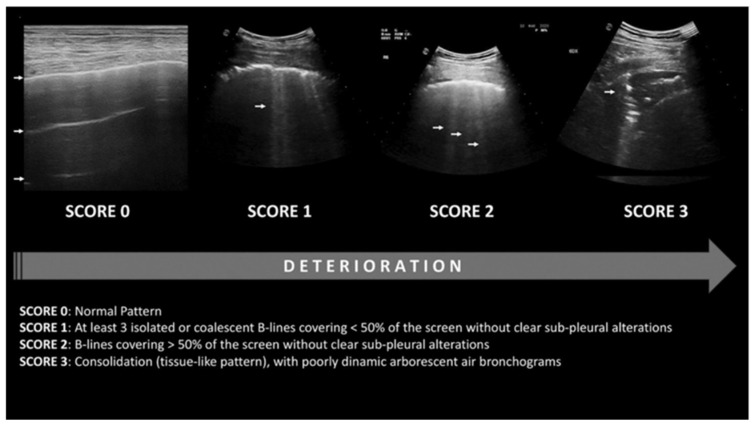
Lung finding for each lung ultrasound score with 12 lung fields. Lung regions exhibiting normal aeration (N) are assigned a score of 0 (white arrows with score 0 denoting A lines); moderate aeration loss (B1) receives a score of 1 (white arrows with score 1 denoting B lines); severe aeration loss (B2) is given a score of 2 (white arrows with score 2 denoting B lines covering > 50% of the screen); and full aeration loss (consolidation; C) is allocated a score of 3 (white arrows denoting consolidation). The cumulative readings for all 12 regions yield a LUS score ranging from 0 to 36. The figure is reproduced from Dell’Aquila et al. 2022 under the Creative Commons Attribution 4.0 License [79].
